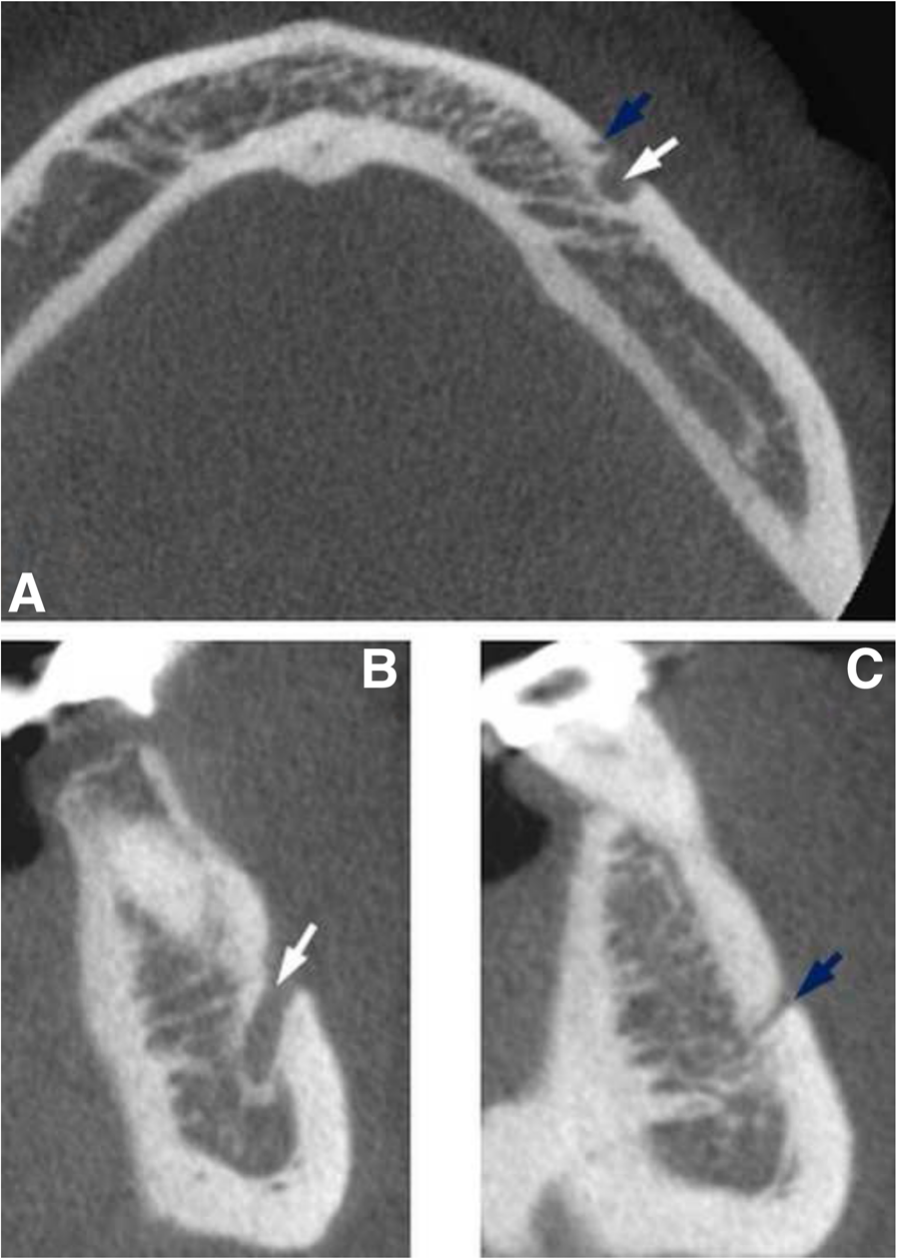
CBCT images of mental and accessory mental foramen. a Axial image. b Cross-sectional image at the mental foramen. c Cross-sectional image at the accessory mental foramen. *White arrowhead* - mental foramen, *blue arrowhead* - accessory mental foramen
